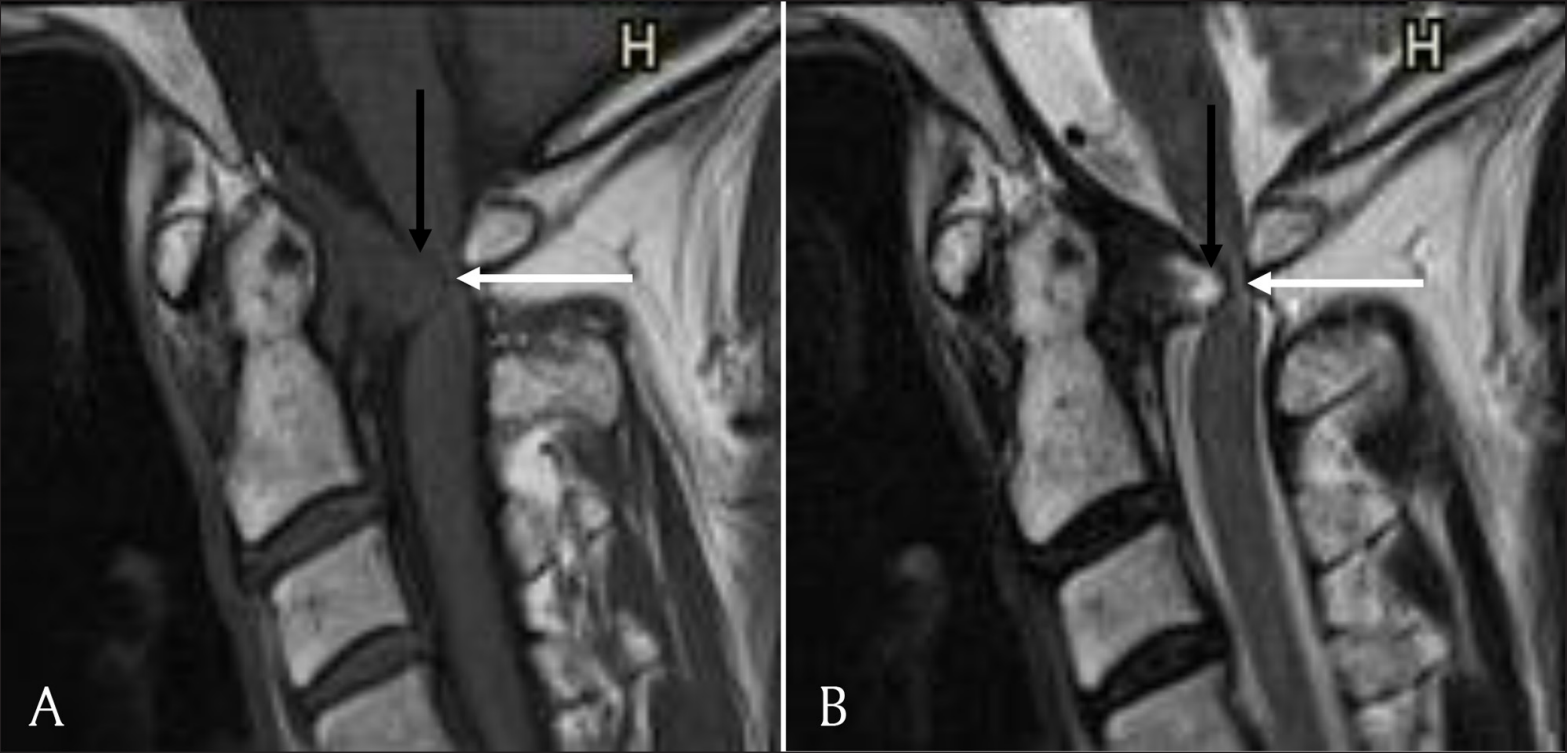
(A) Sagittal T1-weighted image. The retro-odontoid pseudotumor is seen as a mass (black arrow) extruding through the transverse ligament and compressing the myelum (white arrow). It has an isointense signal compared to the myelum. (B) Sagittal T2-weighted image. The retro-odontoid pseudotumor has a hyperintense signal (black arrow) and compresses the myelum (white arrow).
